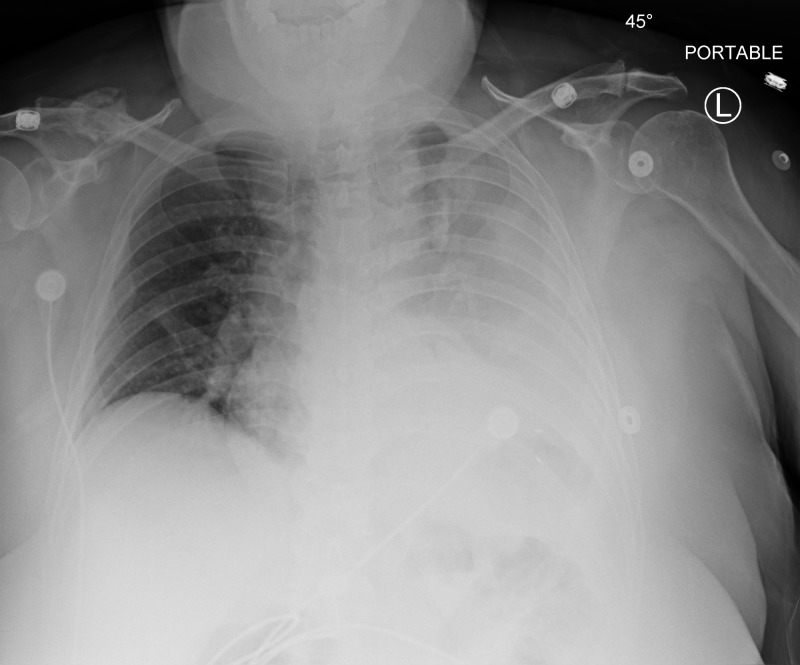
Repeat chest X-ray two days after admission reveals near-complete opacification of the left hemithorax
